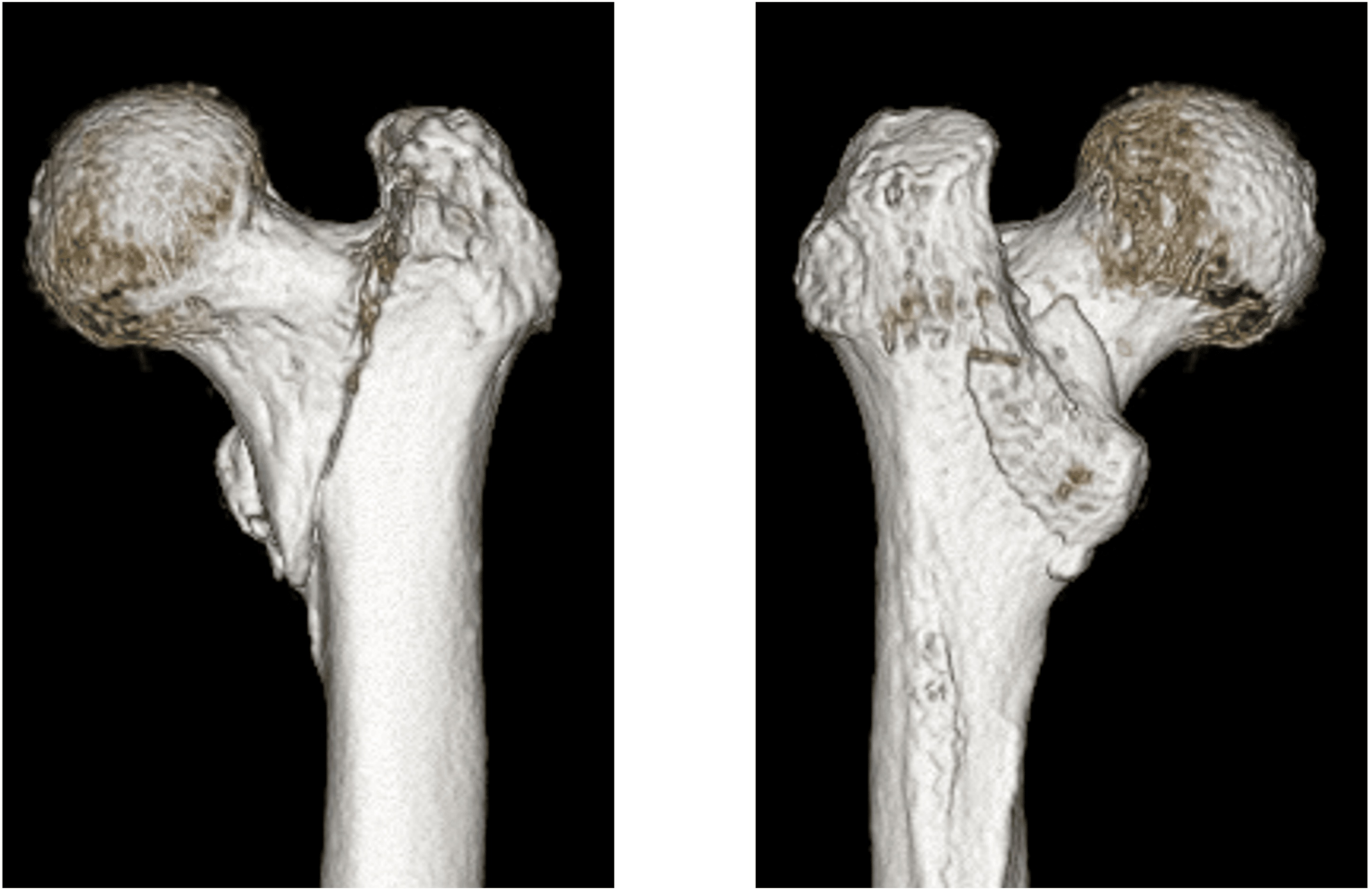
Pre-operative 3D computed tomography (CT) images of left hip. A main fracture line was observed at the intertrochanteric line, and a bone fragment including the lesser trochanter was observed posterior medially.
